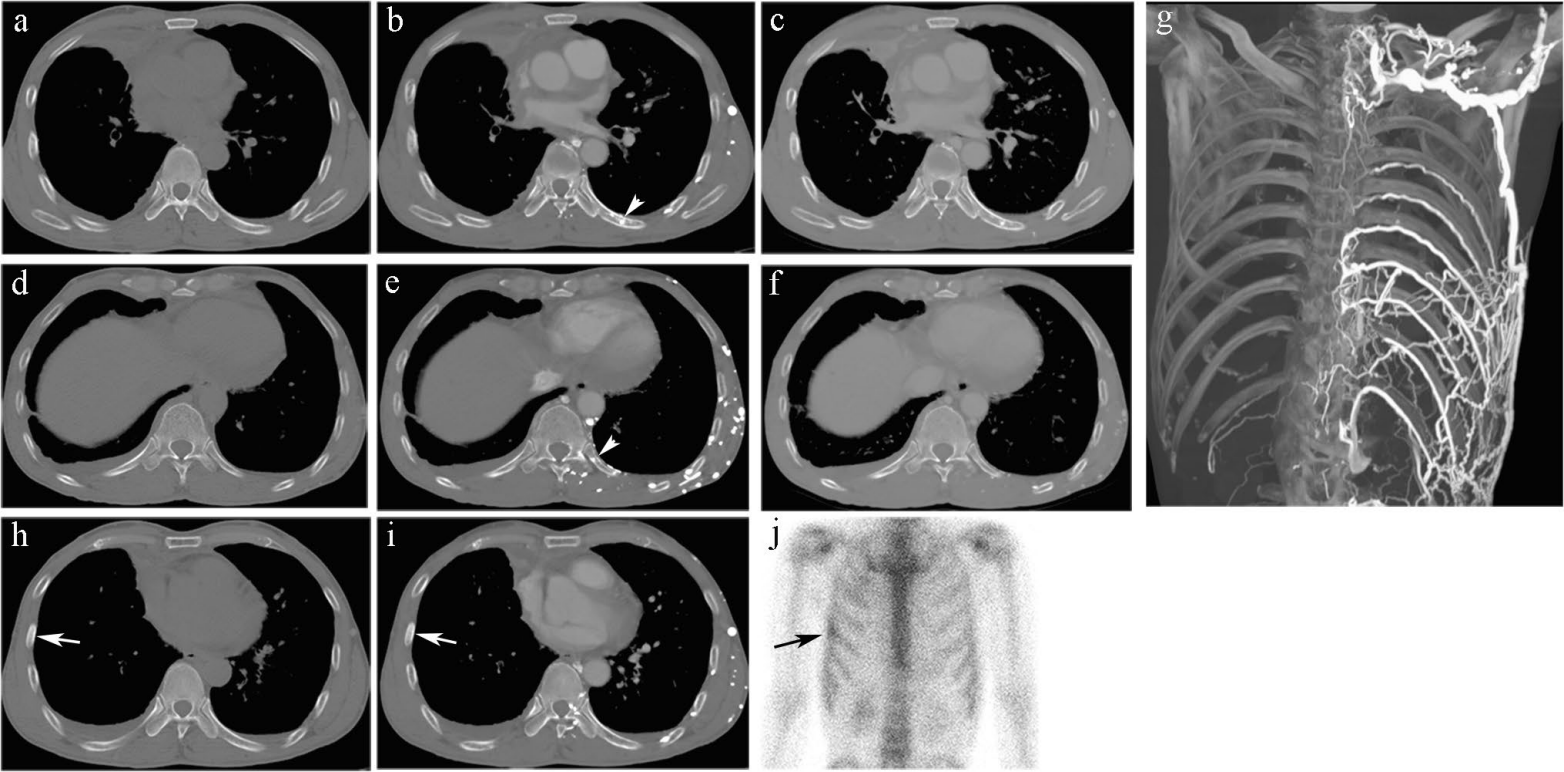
A 53-year-old man with a diagnosis of right central-type lung cancer and right hilar and mediastinal lymph node metastases, complicated by a type 2 CVO. The contrast material was administered via injection into the left arm. The left lower ICVP filled with contrast (b) and communicated with the anterior/lateral thoracic venous plexus (g, Maximum Density Projection). Patchy /nodular REs were seen in the left eighth (b, white arrowhead) and 11th posterior ribs (e, white arrowhead). There was no abnormality on unenhanced scan (a and d) and the degree of enhancement decreased and shrank /disappeared in the venous phase (c and f). Patchy sclerotic metastasis was detected in the right sixth rib (h, unenhanced CT, white arrow; i, arterial phase). The sclerotic metastasis showed radioactivity concentration on ECT (j) performed within an interval of 2 days, while no visible abnormality was detected in the RE regions
